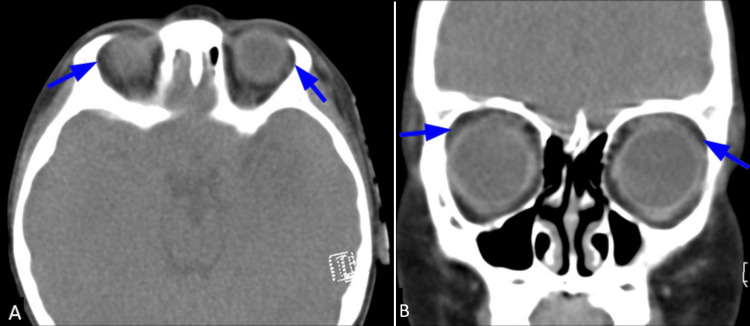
Congenital alacrima A four-year-old boy presenting with a foreign-body sensation and pain in both eyes. Contrast-enhanced computed tomography (CT) of orbits (A: axial; B: coronal sections) reveals bilateral absence of the lacrimal glands (blue arrows).
